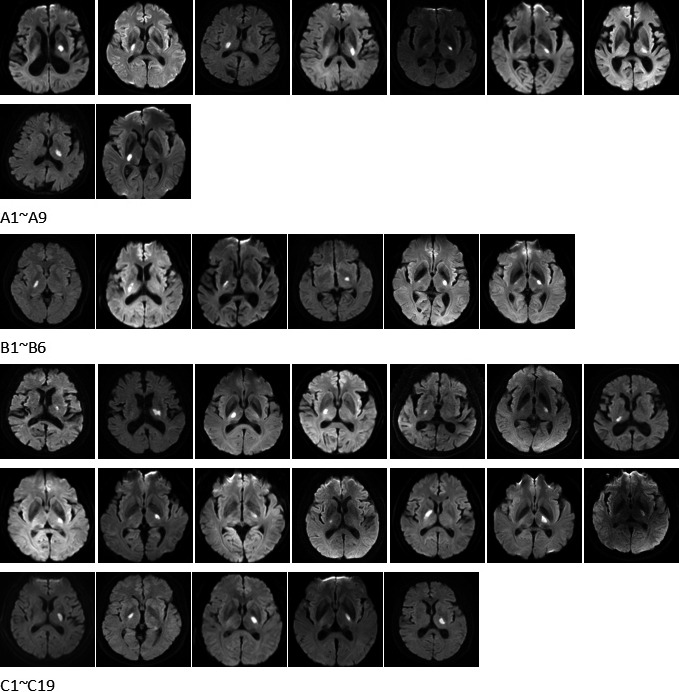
MR images of a total of 34 patients
